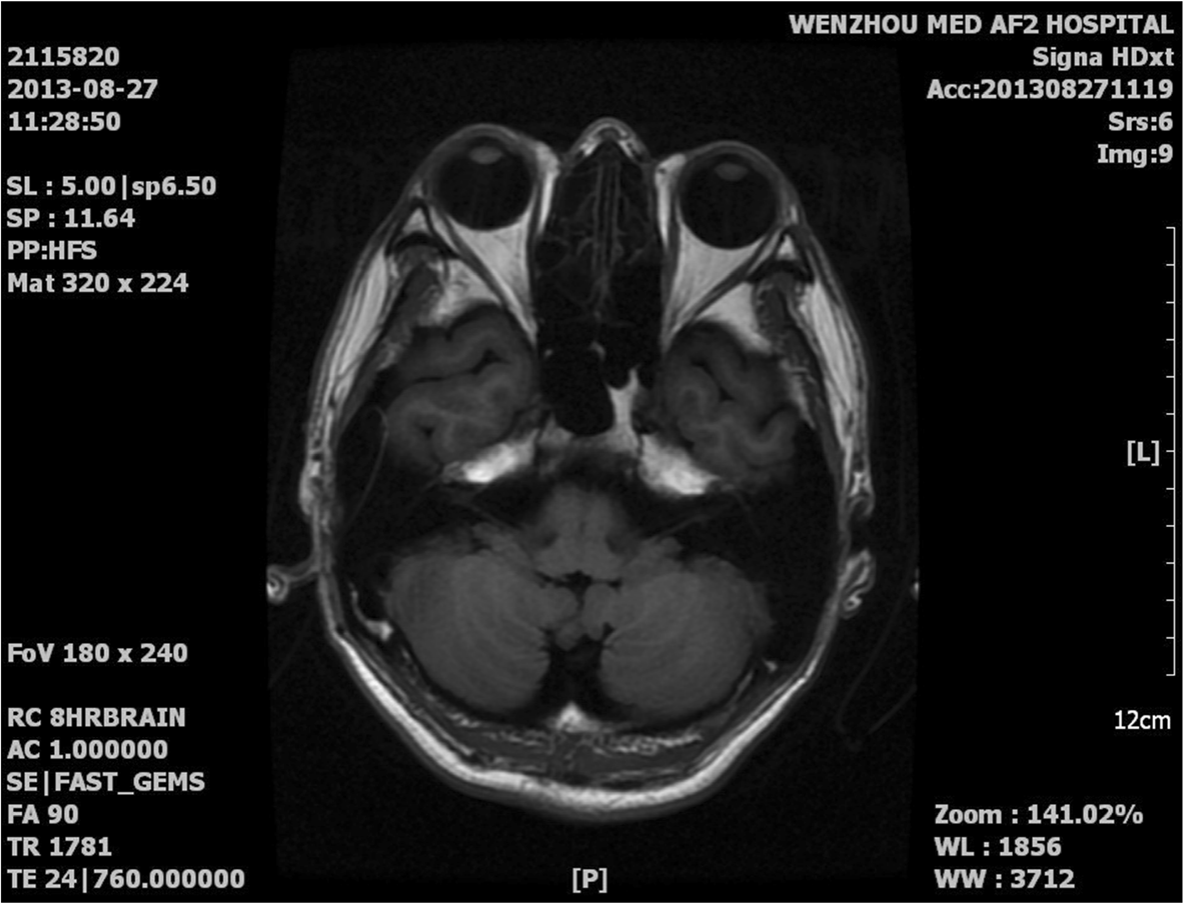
Cranial MRI image of the horizontal position. No occupying lesions or abnormal signal of the inferior olive was observed
